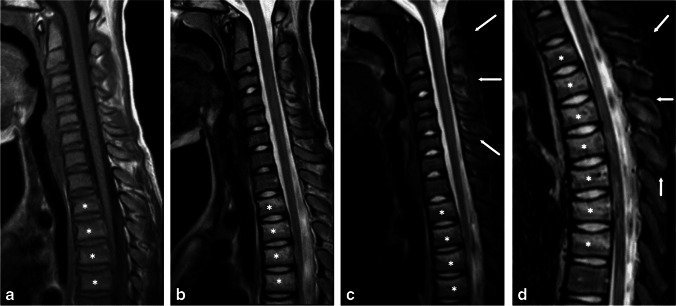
Magnetic resonance images of an 11-year-old boy after a trampoline accident. a–c Sagittal T1 (a), T2 (b) and short tau inversion recovery (STIR) (c) of the cervical spine. d Sagittal STIR of the thoracic spine show compression fractures of the second to eighth thoracic vertebrae (*asterisks*) and edema in the interspinous ligament (*arrows*)
